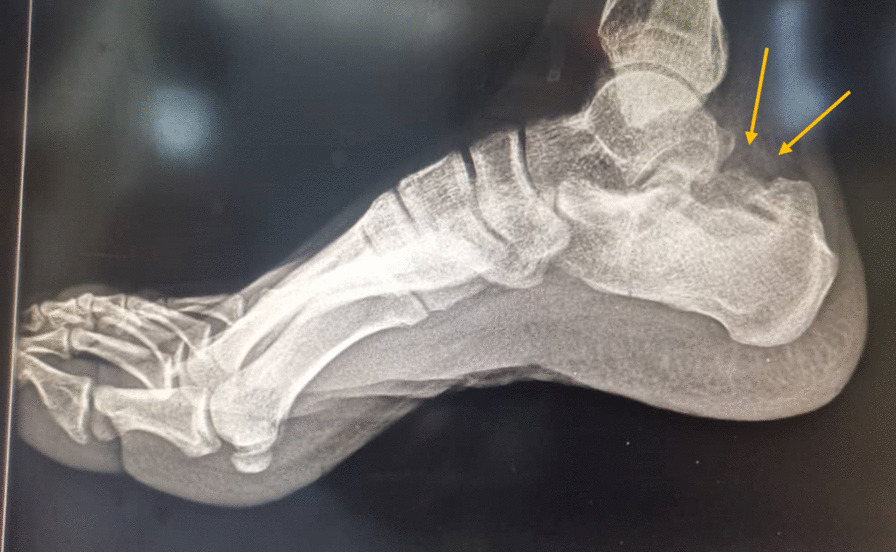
lateral view X-ray Right foot, shows features of cortical erosion and central cavitation consistent with chronic osteomyelitis in the calcaneum bone
